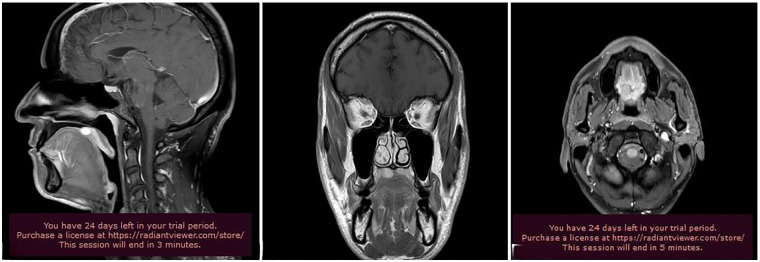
Preoperative magnetic resonance imaging in sagittal (A), coronal (B) and axial planes (C) showing a well-defined palatal soft-tissue lesion without convincing evidence of cortical bone invasion.
